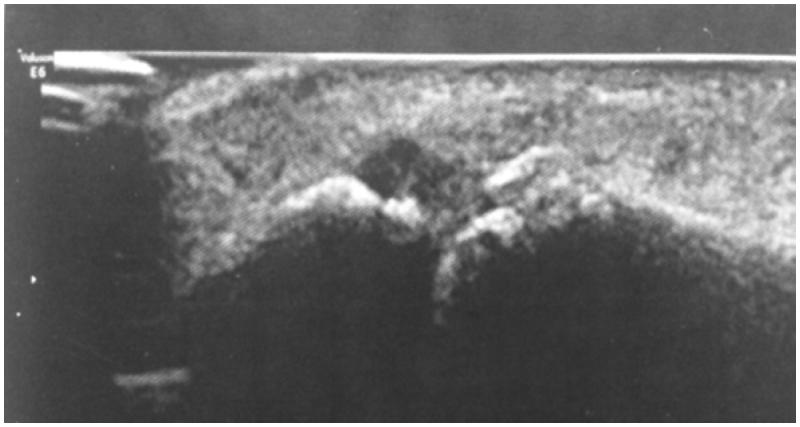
Thumb ultrasound: calcified appearance of MCPL LCL with synovial hypertrophy.
